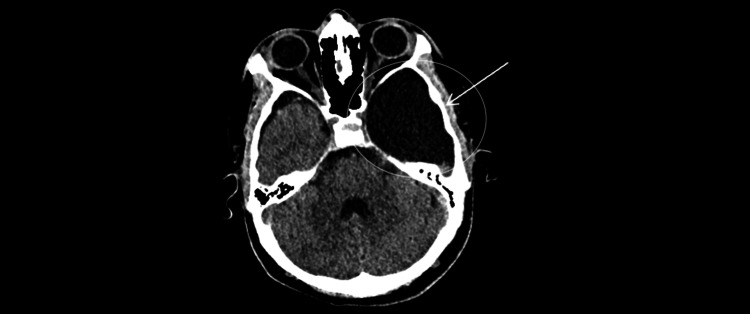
Non-contrast head CT (axial, brain window; W80/L40; 5-mm slices). Large left middle-fossa arachnoid cyst (white arrow) with CSF attenuation, compressing the adjacent temporal lobe; the open circle outlines the region of interest. No acute intracranial hemorrhage is seen at this level.
